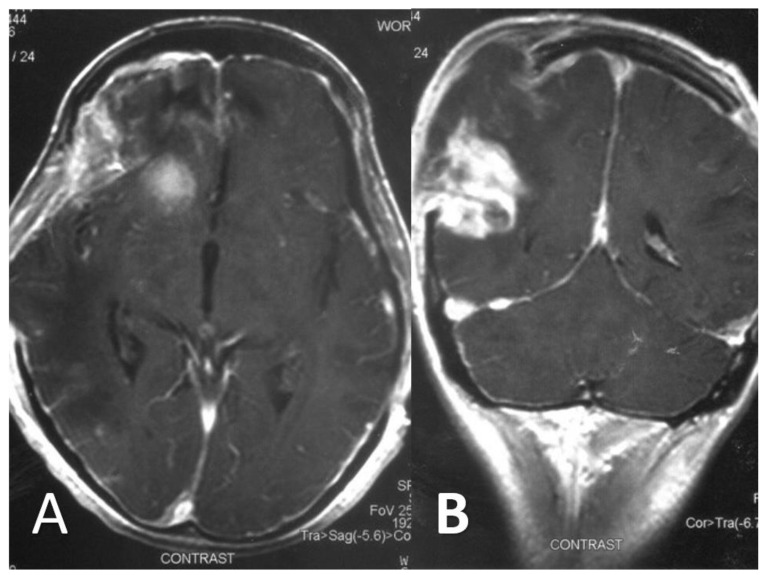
Axial (A) and coronal (B) T1 gadolinium-enhanced MR images following partial surgical debridement showcase a contrast-enhancing round lesion in the right anterior cranial fossa (A), and a heterogeneously enhancing lesion in the right parietal lobe (B). Additionally, markedly increased meningeal enhancement is also depicted in the right cerebral hemisphere (A), suggesting concomitant meningoencephalitis.
